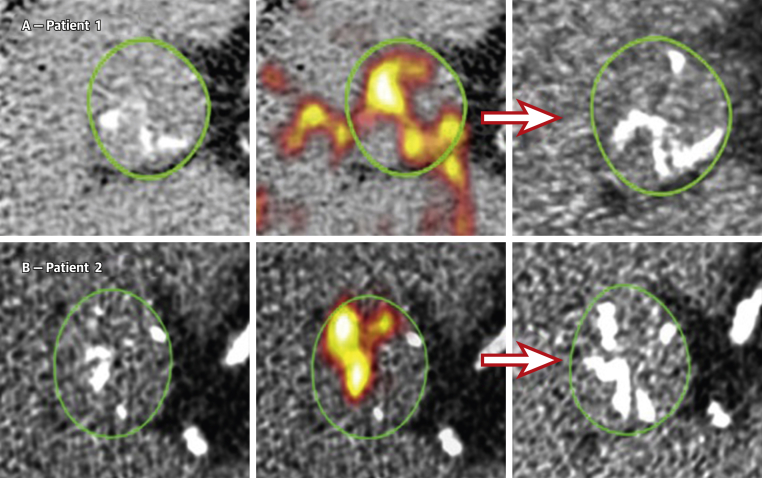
^18^F-NaF PET-CT Predicts Disease Progression in AS Baseline CT calcium score scans (left) for patients 1 (A) and 2 (B). Fused ^18^F-NAF PET-CT scans (middle) show fluoride uptake in red and yellow. Follow-up CT at 1 year (right) suggests that the baseline PET signal predicts where new macroscopic calcium, visible on the CT, is going to develop. Reproduced with permission from Dweck et al. (45). AS = aortic stenosis; other abbreviations as in Figures 1 and 2.
